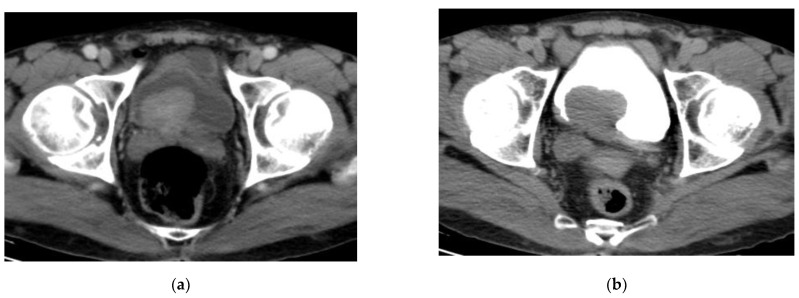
CT urography: (a) nephrographic phase: an enhancing intravesical soft tissue mass at the posterior bladder wall; (b) excretory phase: washout of contrast medium. The bladder mass is recognizable as a filling defect inside the opacified urine. (Urothelial carcinoma, stage T2 N0).
